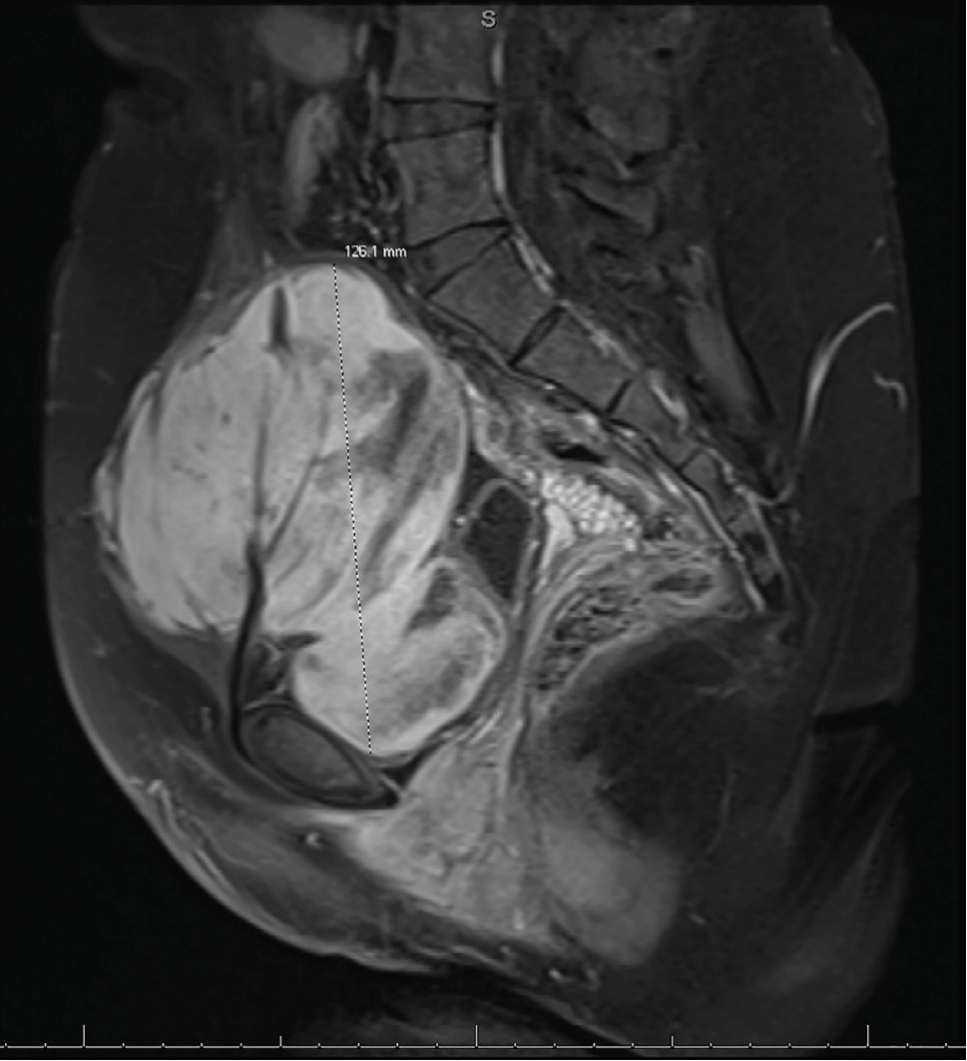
MRI pelvis demonstrating the prominent vascularity supplying the mass, arising from the pubic symphysis region, possibly the inferior epigastric artery.
